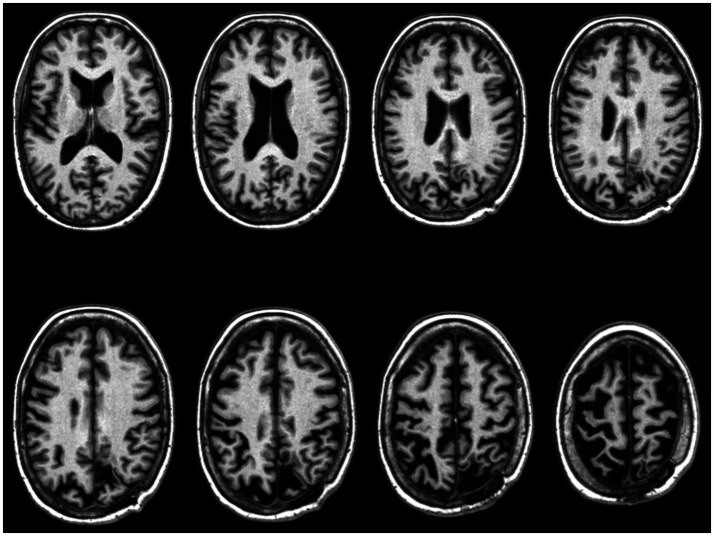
Axial view of CP’s T1-weighted brain scans acquired in 2023 (neurological convention).
